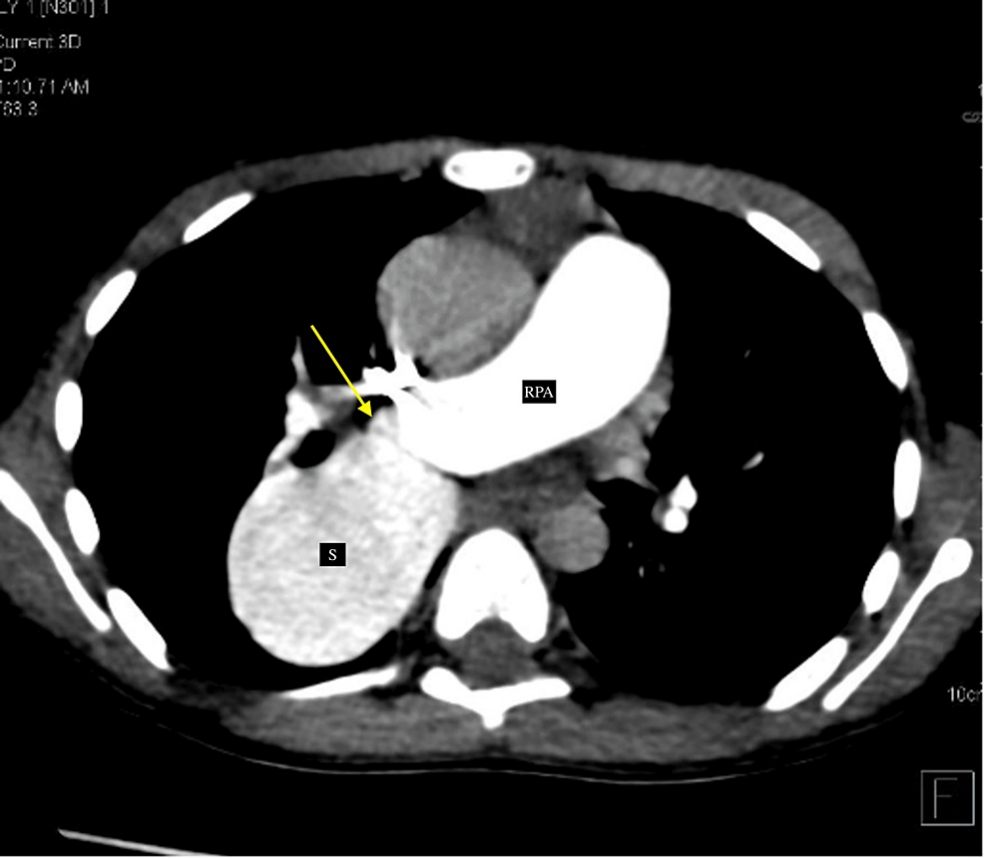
Axial CT reformatted image demonstrating the jet of contrast (yellow arrow) from the RPA in the pulmonary arterial phase into the dilated aneurysmal sac (labeled as "S"). The sac is getting filled in the pulmonary arterial phase, and thus, contrast reaches the left atrium even before the contrast opacification of pulmonary veins. RPA: right pulmonary artery
